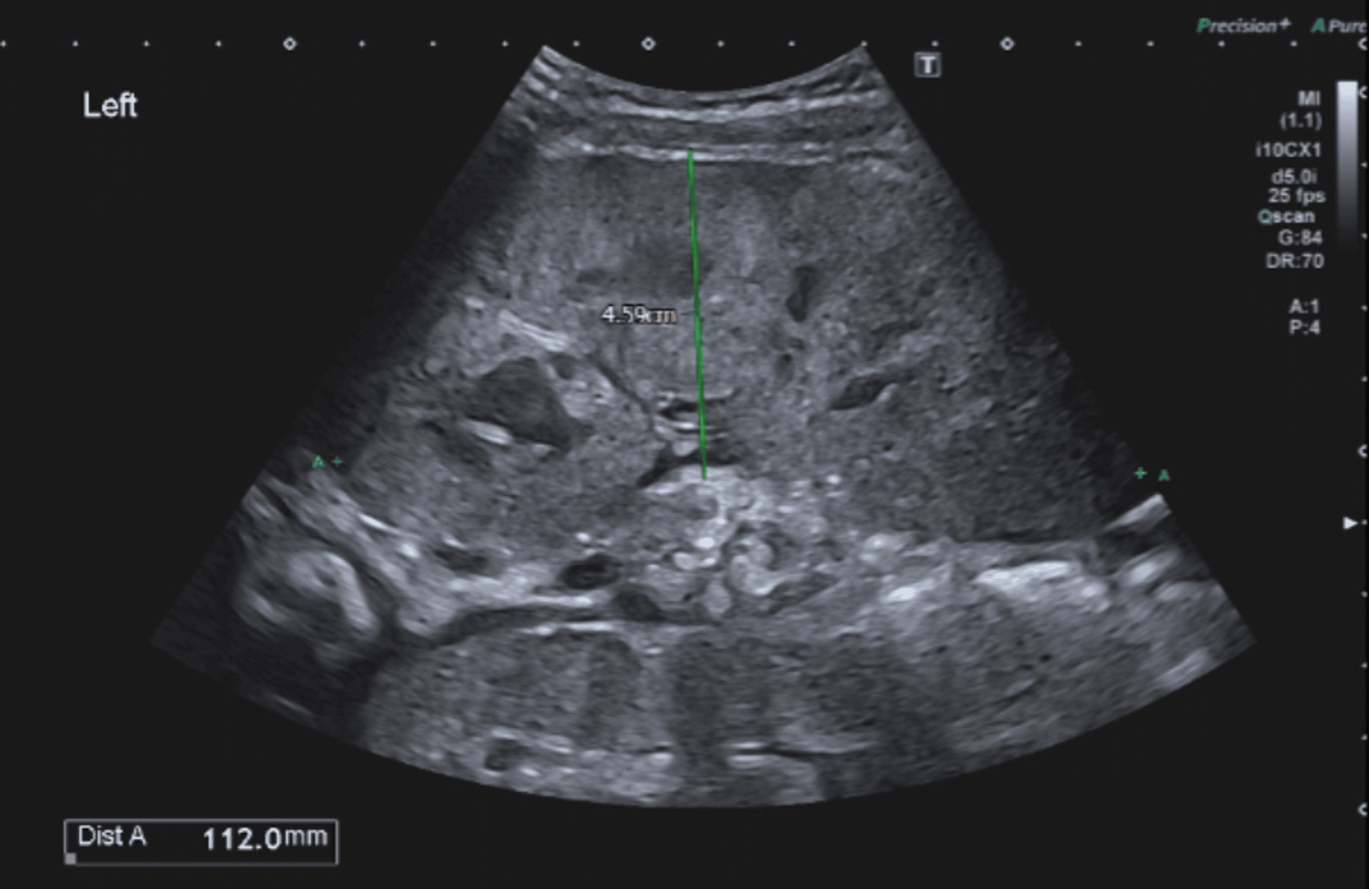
Abdominal ultrasonography of left kidney with largest width measurement of 4.59 cm and kidney length (Dist A) of 11.20cm.
